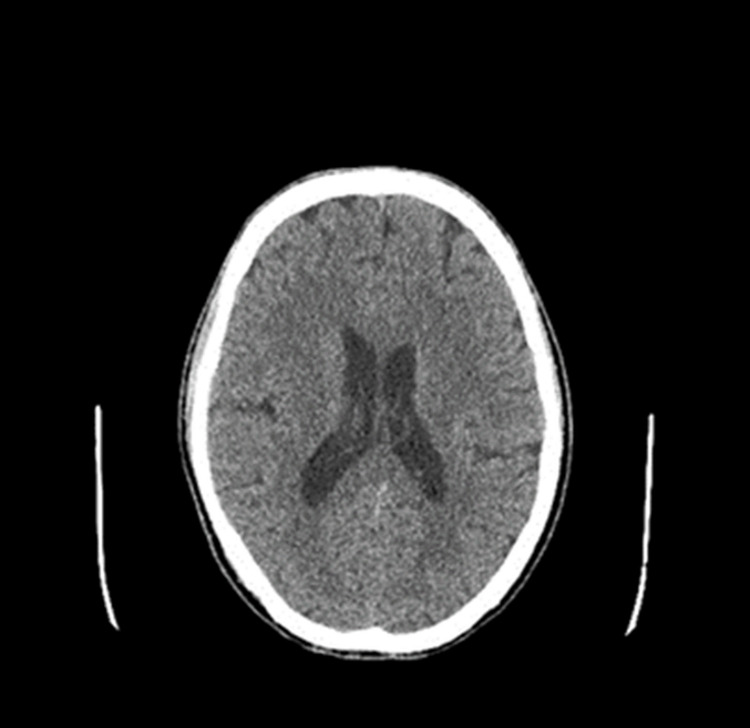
CT scan of the head prior to lumbar puncture showed no hydrocephalus or contraindications to lumbar puncture
